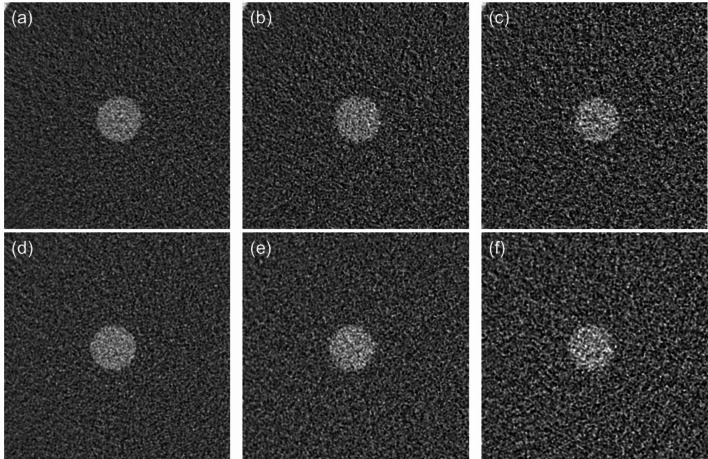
Comparison of virtual monoenergetic images at 70 keV of the rod with 2 mg iodine/mL. These images were obtained at volume CT dose indices of a 15 mGy, b 7.5 mGy, and c 3 mGy for PCD CT; d 15 mGy, e 7.5 mGy, and f 3 mGy for DECT. The window width/level was set at 250/30
